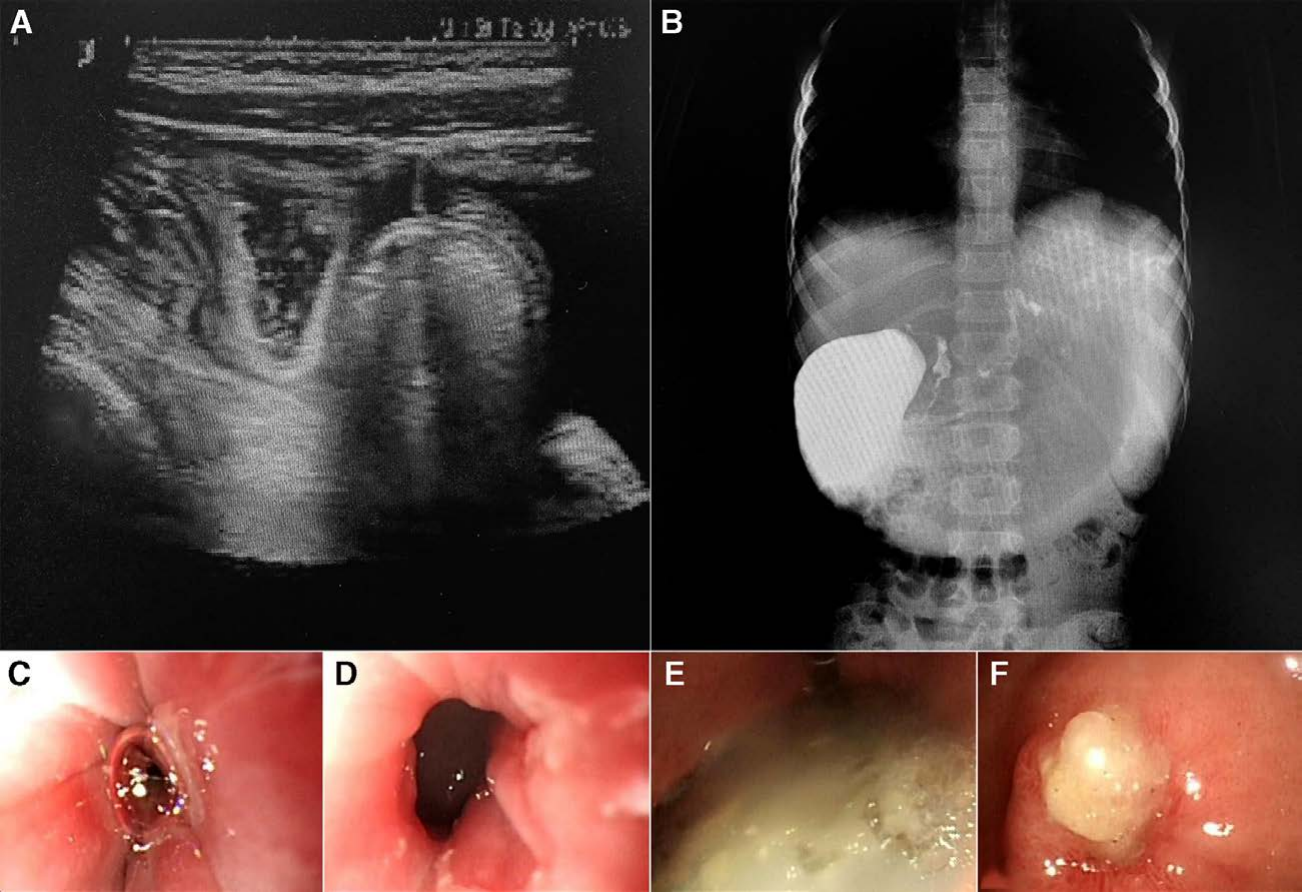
(A) Abdominal ultrasound: more gastric contents were seen in the stomach 30 minutes after eating. (B) Upper gastrointestinal barium meal: the upper gastrointestinal peristalsis was weak, the contrast was difficult to pass through the pylorus, delayed photography, the duodenal circle was poorly displayed, and the proximal jejunum was not visualized. (C–F) Gastroscopy: near the cardia, ulcers covered in white moss are evident. A florid pattern of congestion and edema is present in the stomach sinus. Visible pyloric stenosis is demonstrated with pus-covered ulcers.
